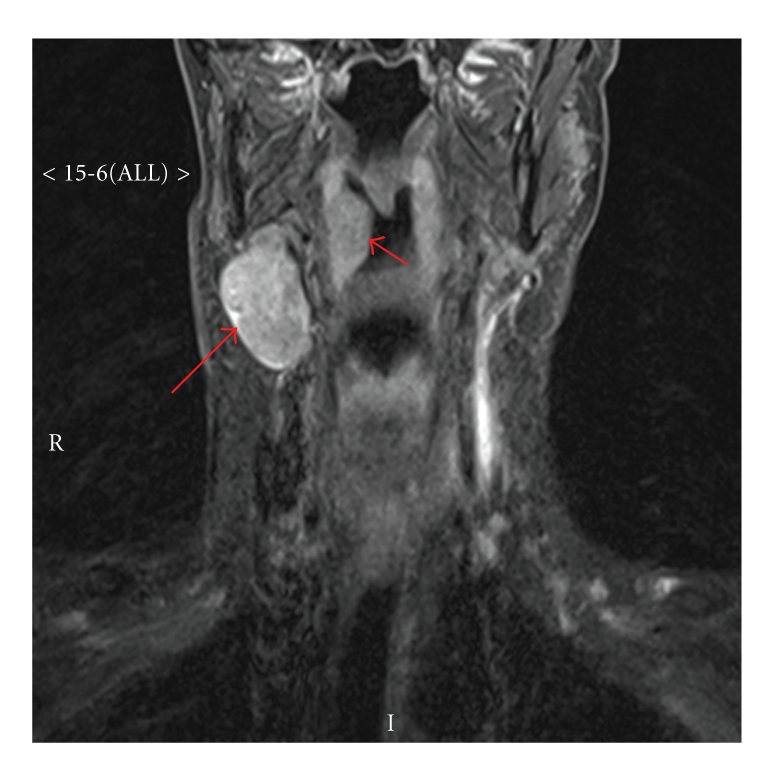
MRI of the neck showing an enlarged lymph node (long arrow) and a bulge in the right tonsillar fossa (short arrow) suspicious for primary tumour.
